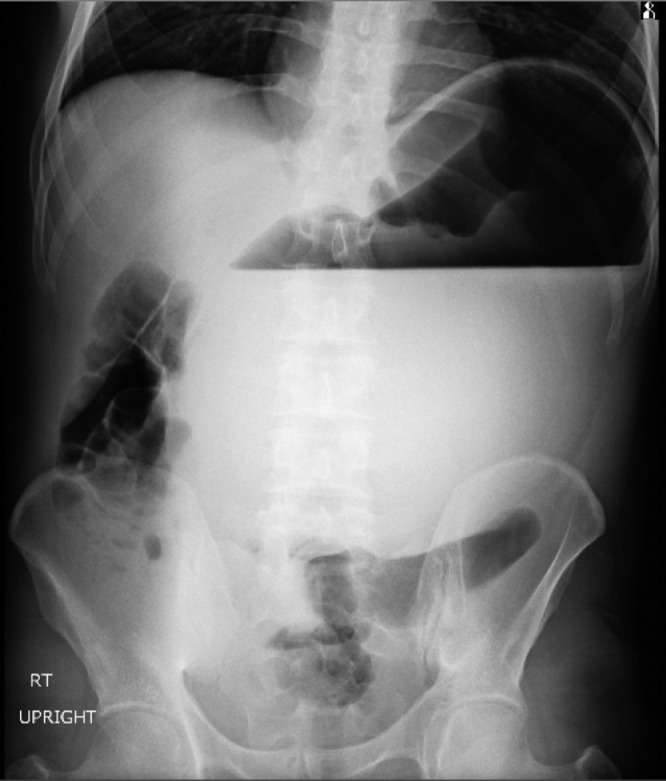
Abdominal X-ray (upright) demonstrates abnormal position of gastric cardia and double bubble gastric air-fluid levels.
